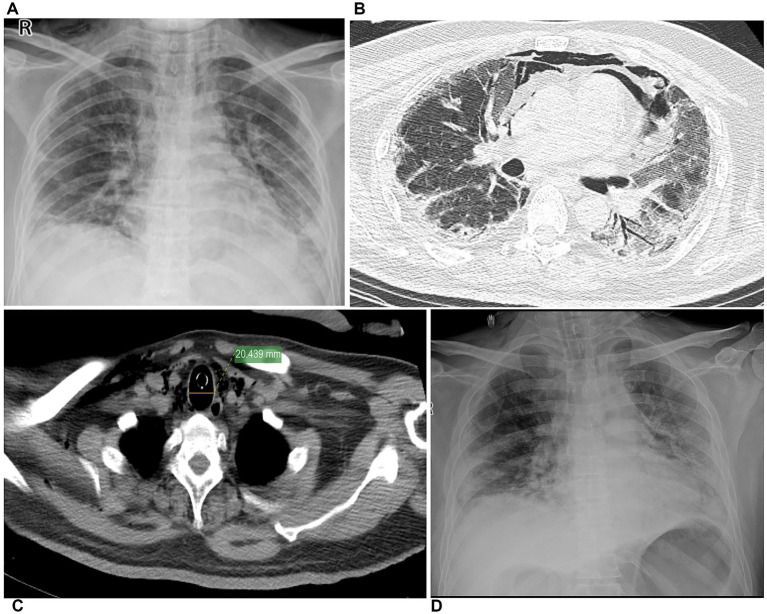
Chest imaging of case 2 (45-year-old male patient, corresponds to typical case 2). (A) Chest X-ray on admission, multiple GGO, and patchy infiltrates, more prominent in the left lung, and minimal air was observed in mediastinum. (B) The lung window revealed multiple air pockets in the mediastinal space and cervical roots, while the lung window showed multiple patchy, mottled, and GGO predominantly in the peripheral regions of both lungs. (C) Mediastinal window showing endotracheal tube and tracheal dilation. (D) On the second day post-admission, X-ray revealed multiple exudative lesions in both lungs, with a slightly more extensive distribution compared to previous imaging. Minimal air was observed in both cervical roots and mediastinum.
